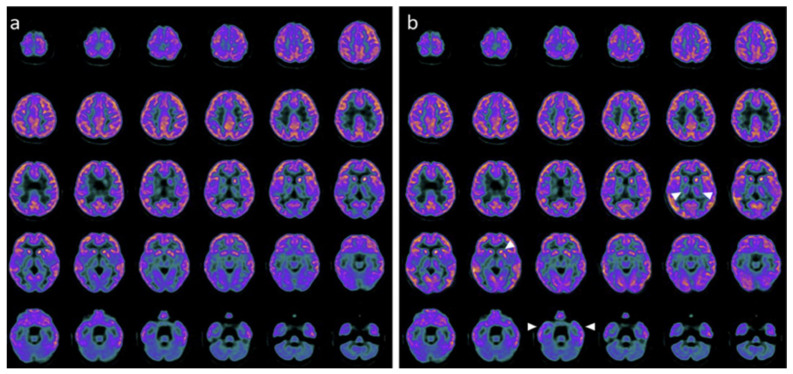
PET before and after the treatment with hr-NGF in the first TBI patient. (a,b) Brain 18F-FDG PET axial slices performed before (a) and after (b) intranasal hr-NGF treatment. A mild global reduction in 18F-FDG uptake was observed in all cortical regions, whereas a more marked reduction was detected in all subcortical regions (a). After hr-NGF administration, an increase in radiotracer uptake was found in the bilateral temporal cortex (right: +7%; left: +7%), right and left thalamus (+6% and +4%, respectively), and the left caudate nucleus (+9%). The white arrows delineate the aforementioned areas. (b).
